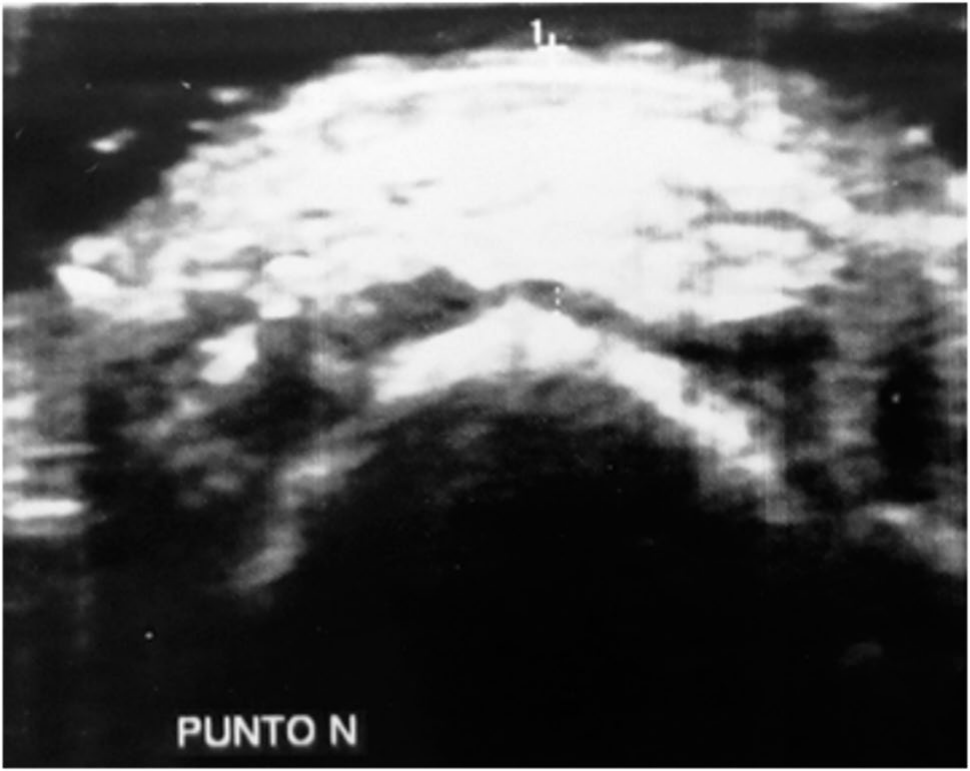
Ultrasonographic soft tissue thickness over N cephalometric point
